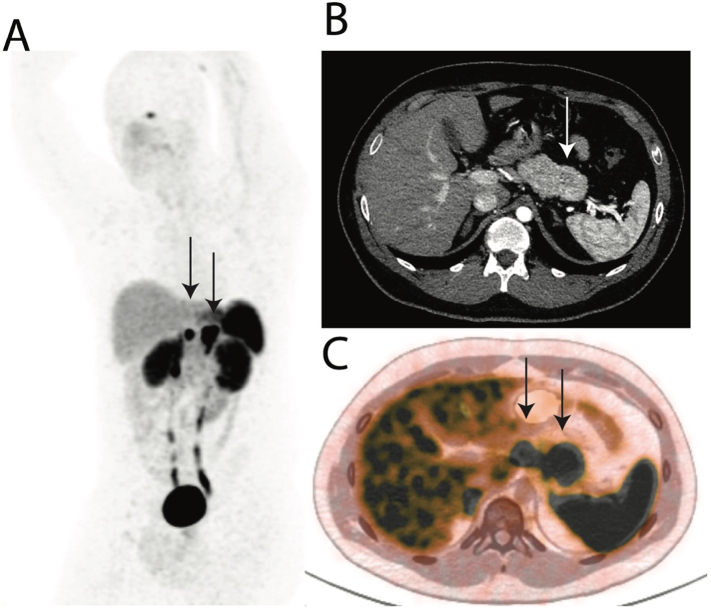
Pre-operative imaging showing two presumed pNETs within pancreatic body and tail. (A) Max Intensity Projection (MIP) of Ga68-DOTATATE PET/CT showing coronal projection of pancreatic body and tail lesions indicated by black arrows. (B) Late arterial-phase CT scan with only pancreatic tail lesion identified. (C) Fused 68Ga-DOTATE PET/CT identifying pancreatic body and tail lesions as seen on MIP.
